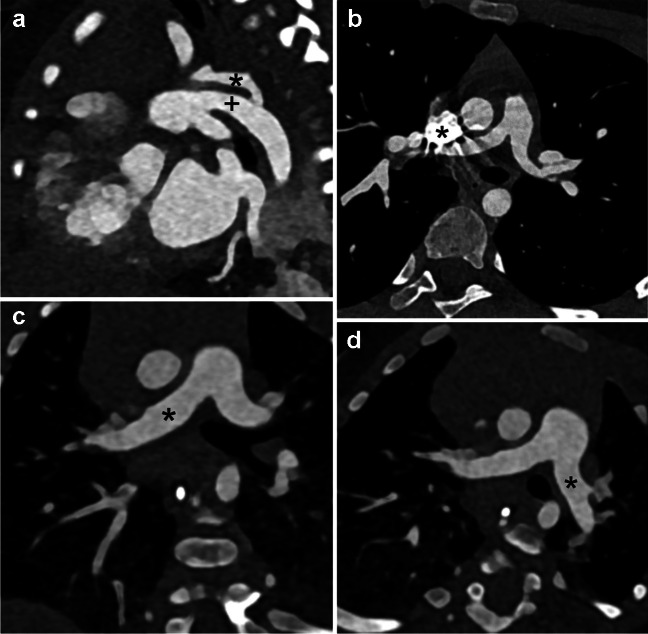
Examples of contrast-enhanced photon-counting computed tomography (PCCT) images using 90 kV protocols. a Sagittal oblique image with a slice thickness of 0.6 mm in an 8-day-old girl with suspected coarctation of the aorta by echocardiography. PCCT shows a hypoplastic arch (*asterisk*) with hypoplasia of the aortic isthmus and a large arterial duct (*plus sign*). This case had a median overall diagnostic score of 4 and a median specific diagnostic score of 4 with a size-specific dose estimate of 0.63 mGy and an effective dose of 0.22 mSv. b Transaxial image with a slice thickness of 0.6 mm in an 8-year-old girl with arterial vasculopathy, status post dilatation of the pulmonary arteries and the ascending aorta due to stenosis. Note the streak artefact from the relatively dense contrast medium (*asterisk*). Despite this, the pulmonary arteries and the ascending aorta were sufficiently visualised for diagnostic purposes. This case had a median overall diagnostic score of 3 and a median specific diagnostic score of 4 with a size-specific dose estimate of 1.09 mGy and an effective dose of 0.24 mSv. c, d Coronal (c) and transaxial (d) oblique images with a slice thickness of 0.6 mm depict the right (c; *asterisk*), and left (d; *asterisk*) pulmonary arteries in a 5-month-old prematurely born girl with idiopathic pulmonary arterial hypertension. The pulmonary arteries were well visualised without stenosis. This case had a median overall diagnostic score of 3 and a median specific diagnostic score of 3 with an effective dose of 0.30 mSv
